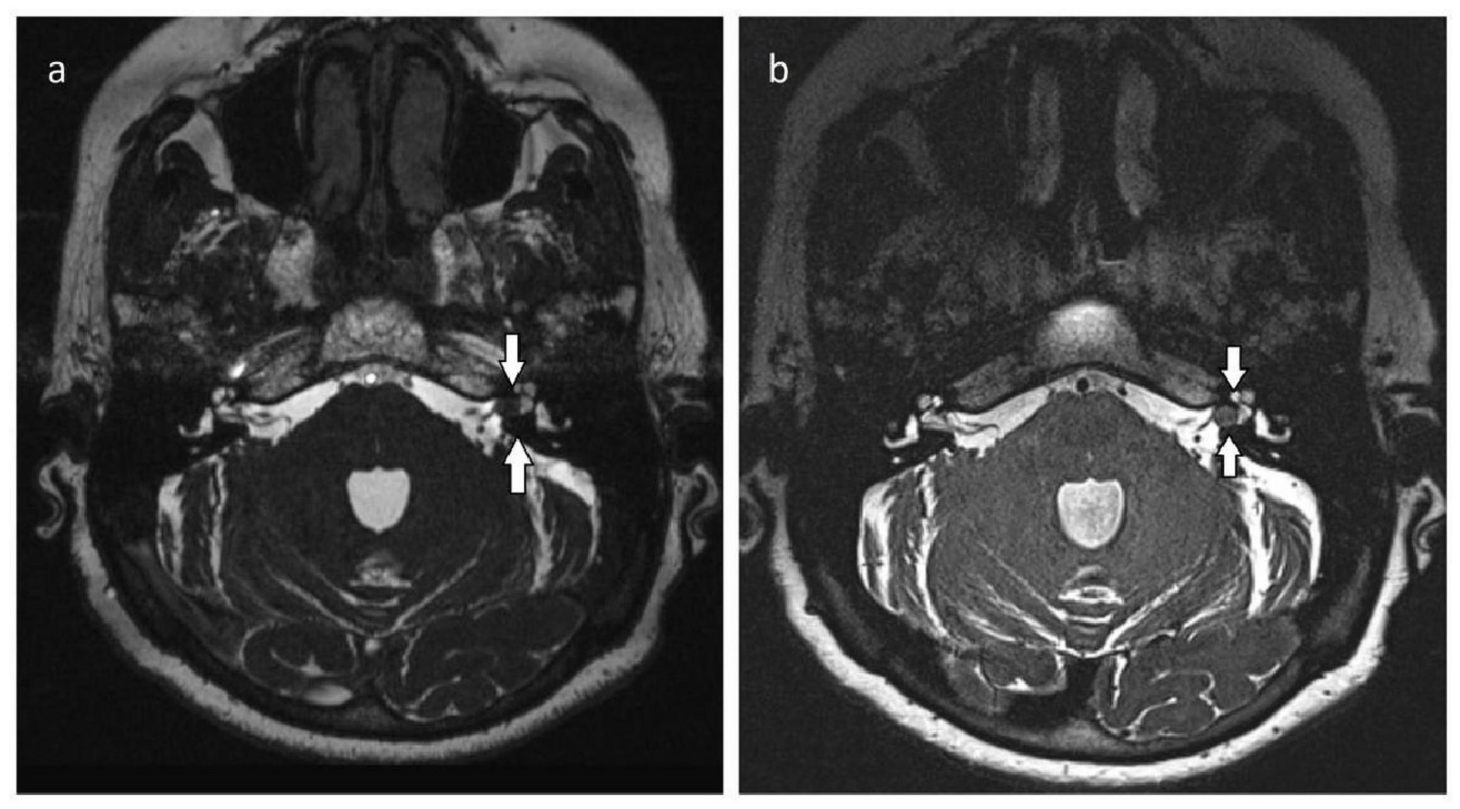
Axial T2-weighted 3D-CISS (a) and (b) 3D-SPACE images of vestibular schwannoma within the left internal auditory canal. Comparison of 3D-CISS and 3D-SPACE sequences. The border between the lesion and adjacent bone is more clearly visualized with 3D-SPACE images (arrows).
